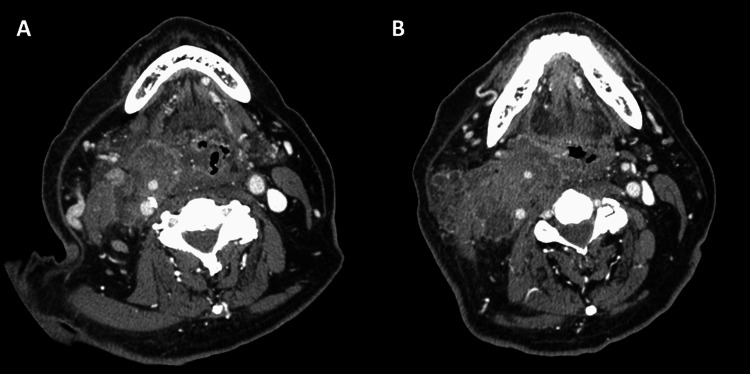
Contrast-enhanced CT of the neck. (A) and (B) Contrast-enhanced CT: a 65-mm-long bifurcated mass was found mainly in the right carotid artery space. The margins are irregular, and the contrast effect is heterogeneous. The right common carotid artery bifurcation to the internal and external carotid arteries is involved in the mass. CT: computed tomography.
